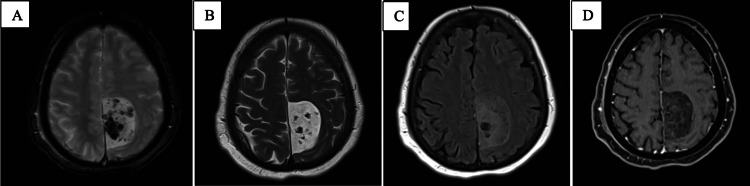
This figure comprises four MRI sequences. A: T2-weighted MRI; B: T2-weighted contrast MRI; C: fluid-attenuated inversion recovery (FLAIR) MRI; and D: T1-weighted contrast MRI. The MRI scans reveal a left frontoparietal parafalcine extra-axial lesion, measuring approximately 4.9 cm (anteroposterior, AP), 3.1 cm (transverse, TR), and 4.6 cm (craniocaudal, CC). This lesion exhibits heterogeneous signal intensities on T1 and T2/FLAIR sequences, incorporating multiple intrinsic T1 hyperintense foci that correspond to hemorrhagic densities previously observed on a CT scan. These findings are accompanied by gradient echo (GRE) bloom artifacts, heterogeneous signals on diffusion-weighted imaging (DWI), and mild to moderate heterogeneous enhancement on postcontrast sequences.
